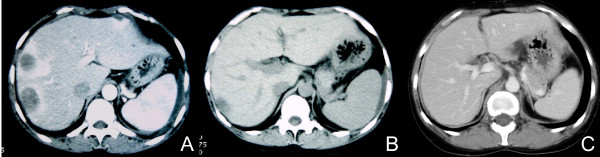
Computed tomography before (A), four weeks after (B), and three months after (C) reduction of immunosuppression remarkably reduced that liver involvement.
